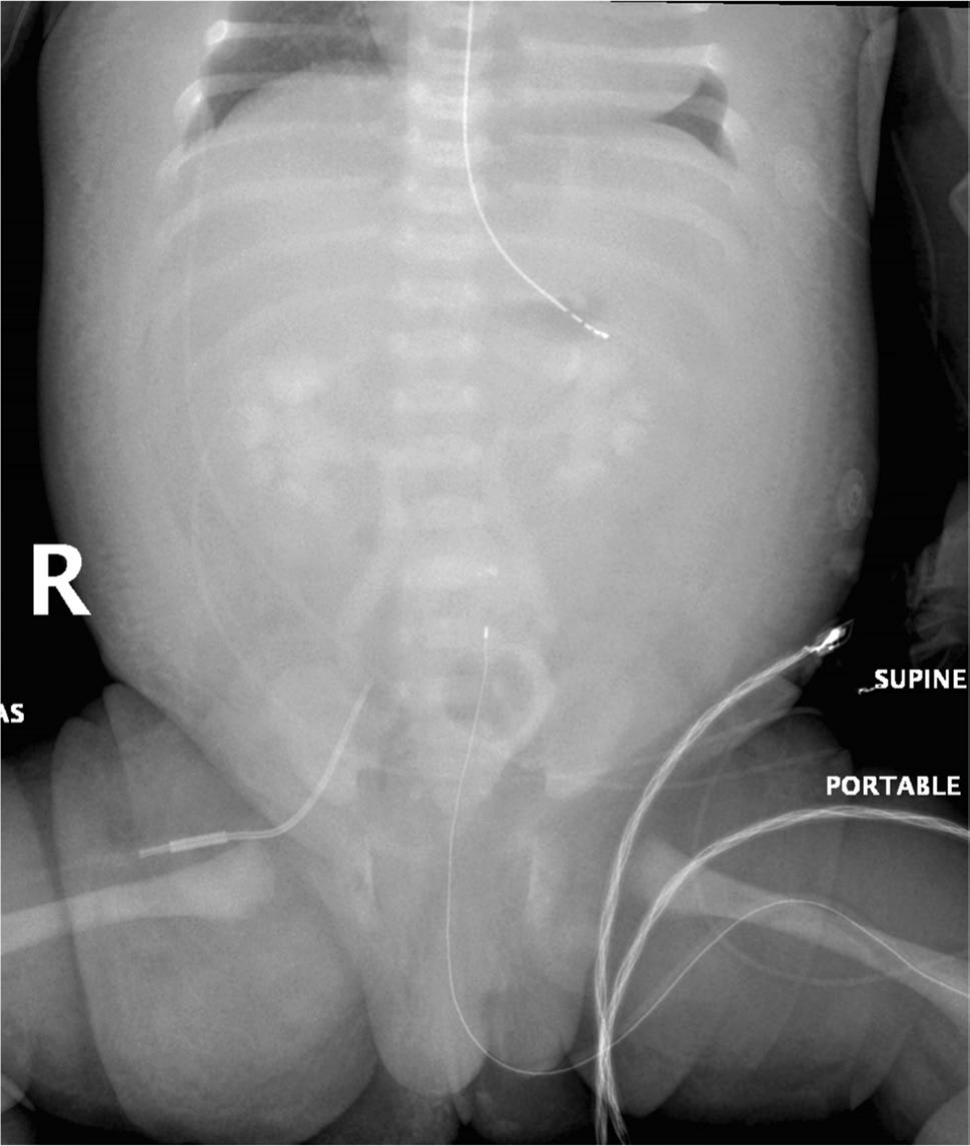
Abdominal radiograph of a 7-day-old girl with double outlet right ventricle and total anomalous pulmonary venous return who underwent cardiac catheterization 84 min prior. The radiograph demonstrates relative enhancement of the subcutaneous tissue and serosal surfaces, causing the entire abdominal cavity to appear relatively lucent
